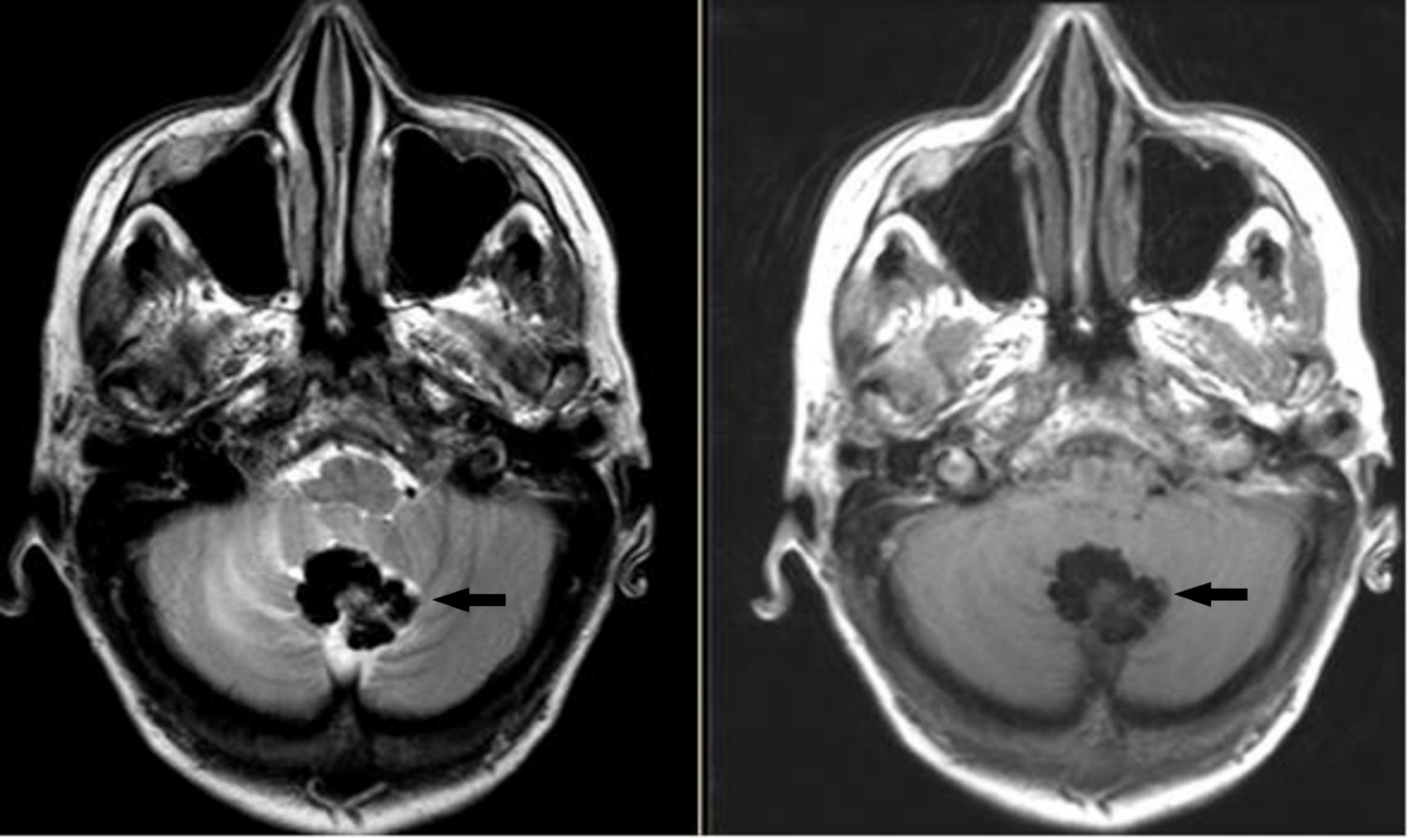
Preoperative MRI T2 (left) and T1 (right) Axial MRI images. Black arrows show the CAPNON. CAPNON: Calcifying pseudoneoplasms of the neuraxis; MRI: Magnetic resonance imaging.
